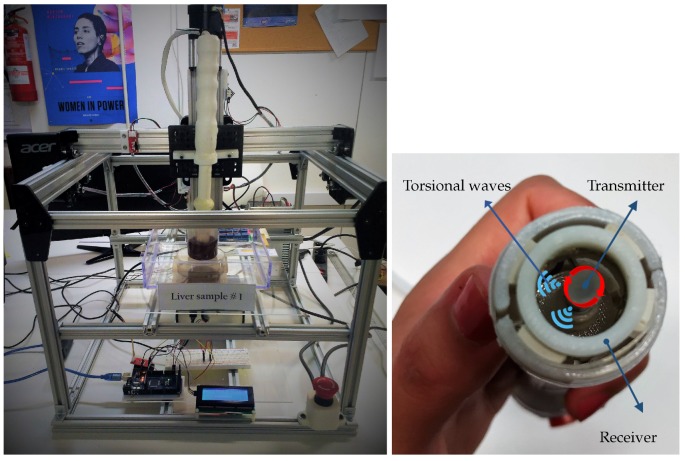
Set-up for measurements using TWE technique. The picture was taken during the measurements at the Ultrasonics Lab at the University of Granada. The figure on the left is a computer numerical control (CNC) system for positioning and pressure-control of the TWE probe. The right figure shows a cross-section of the TWE probe.
